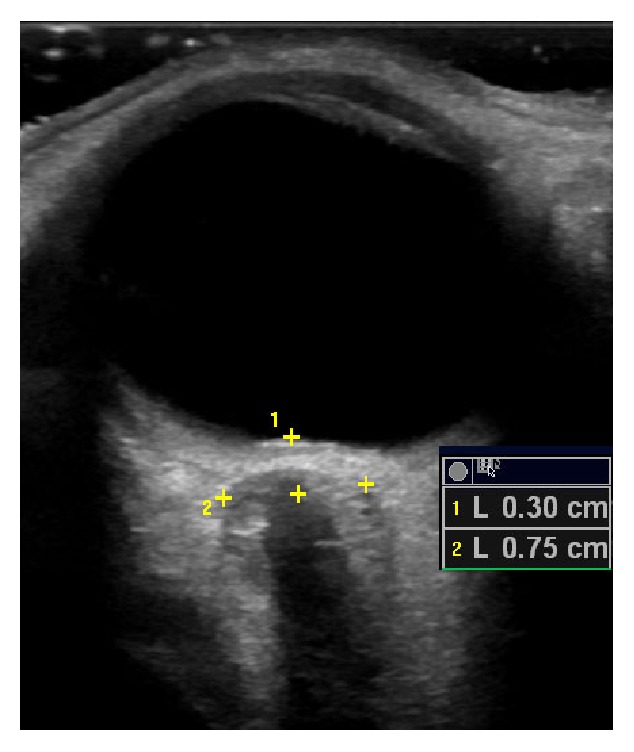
Showing an ultrasound scan with an ONSD of 7.5 mm measured 3 mm posterior to the globe.
